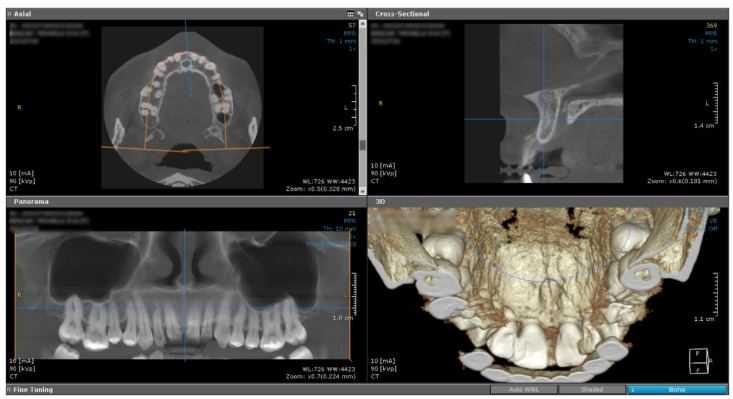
Cone beam computed tomography showed no underlying bone structure alteration.
